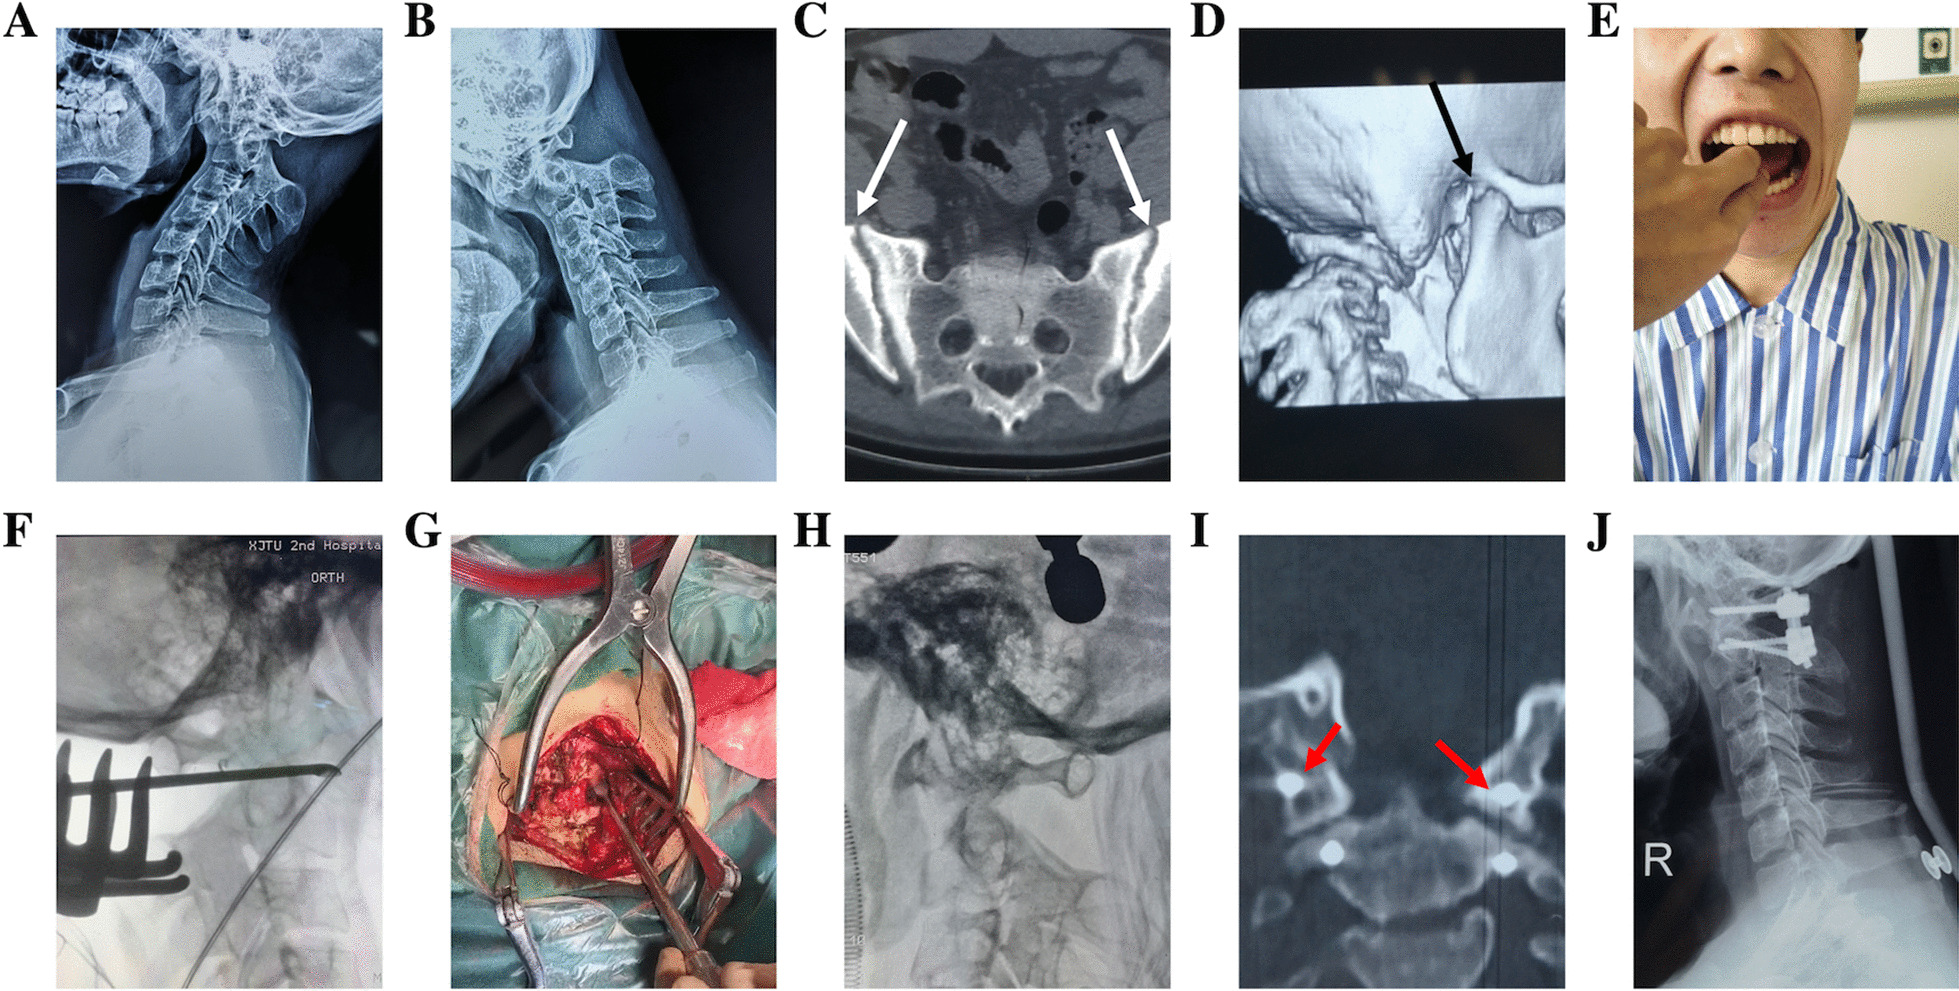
A 23-year-old male patient was admitted for neck pain with numbness of both upper limbs for 8 months. The ADI was 7.41 mm (a) on preoperative hyperflexion X-ray and 5.32 mm (b) on hyperextension X-ray, and dynamic radiographs showed failed reduction of atlantoaxial dislocation. The patient had ankylosing spondylitis (c, white arrow) and difficulty opening his mouth (e) due to temporomandibular joint fusion (d, black arrow). After anesthesia, the atlantoaxial dislocation could not be completely reduced by skull traction with a weight of 1/6 of body weight, but reduction exceeding 50% was classified as type III. which was converted to type II by translateral mass release (f–h), followed by posterior fixation and fusion. After operation, the lateral mass was completely released (i, red arrow). Postoperative lateral X-rays of the cervical spine showed effective reduction and fixation (j)
